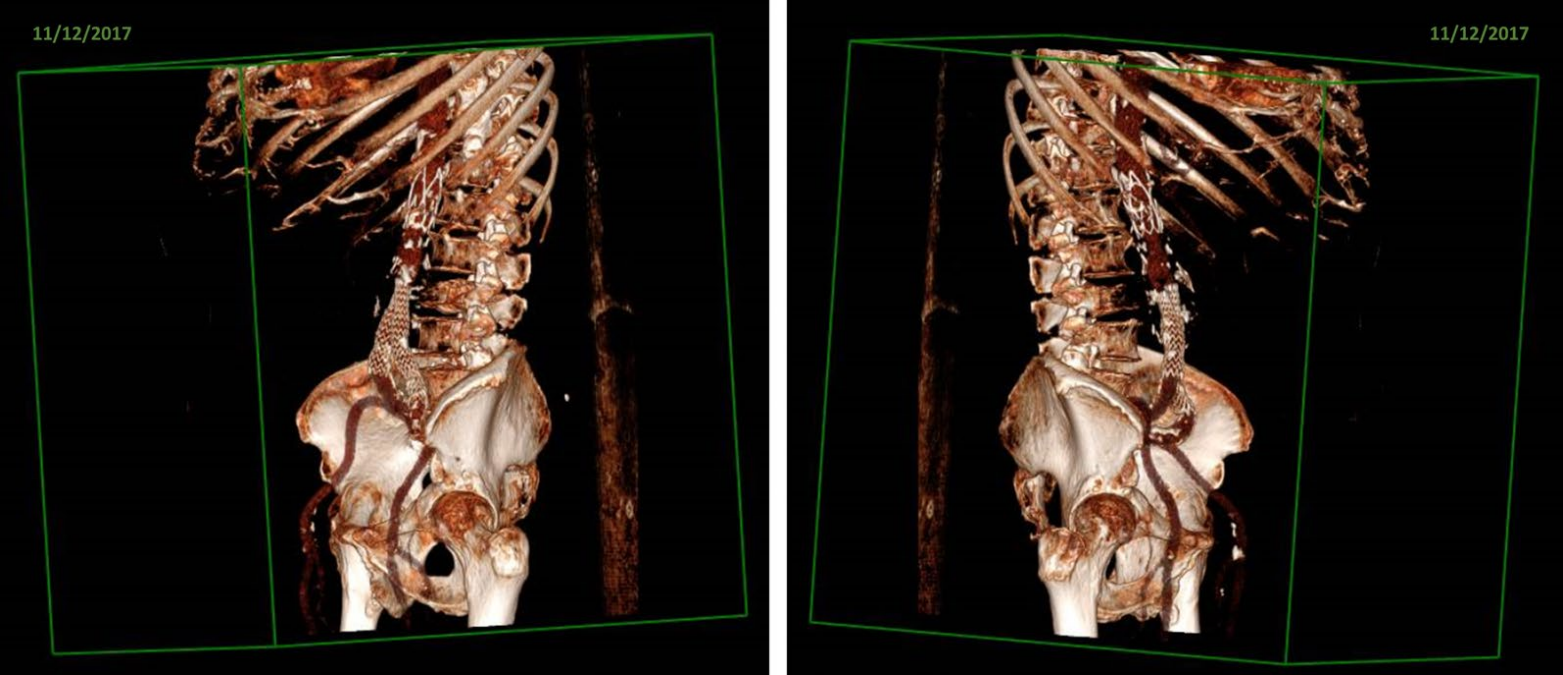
Volume rendering CT reconstruction showing the endoprosthesis
